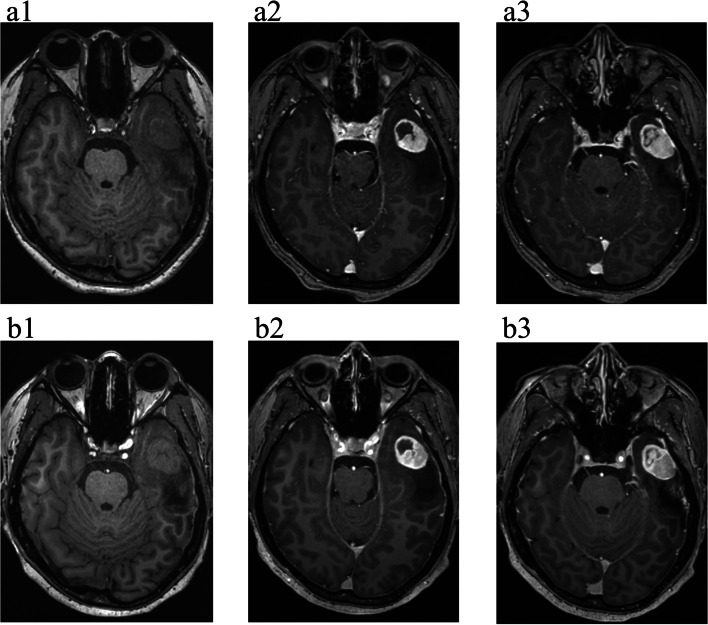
Atypical meningioma, WHO Grade II, 35-year-old male. (a1) T1w MRI without contrast enhancement at 3.0 T and (b1) T1w MRI without contrast enhancement at 5.0 T. (a2) and (a3) Two different slices of full-dose enhanced T1w MRI at 3.0 T. (b2) and (b3) Two different slices of half-dose enhanced T1w MRI at 5.0 T. A 5.0 T MRI displayed a clearer boundary and internal structure of the lesion and showed a stronger inhibitory effect than did 3.0 T MRI; at the same time, in the half-dose 5.0 T images, the degree and range of edge enhancement in the lesion were stronger than those in the full-dose 3.0 T images
